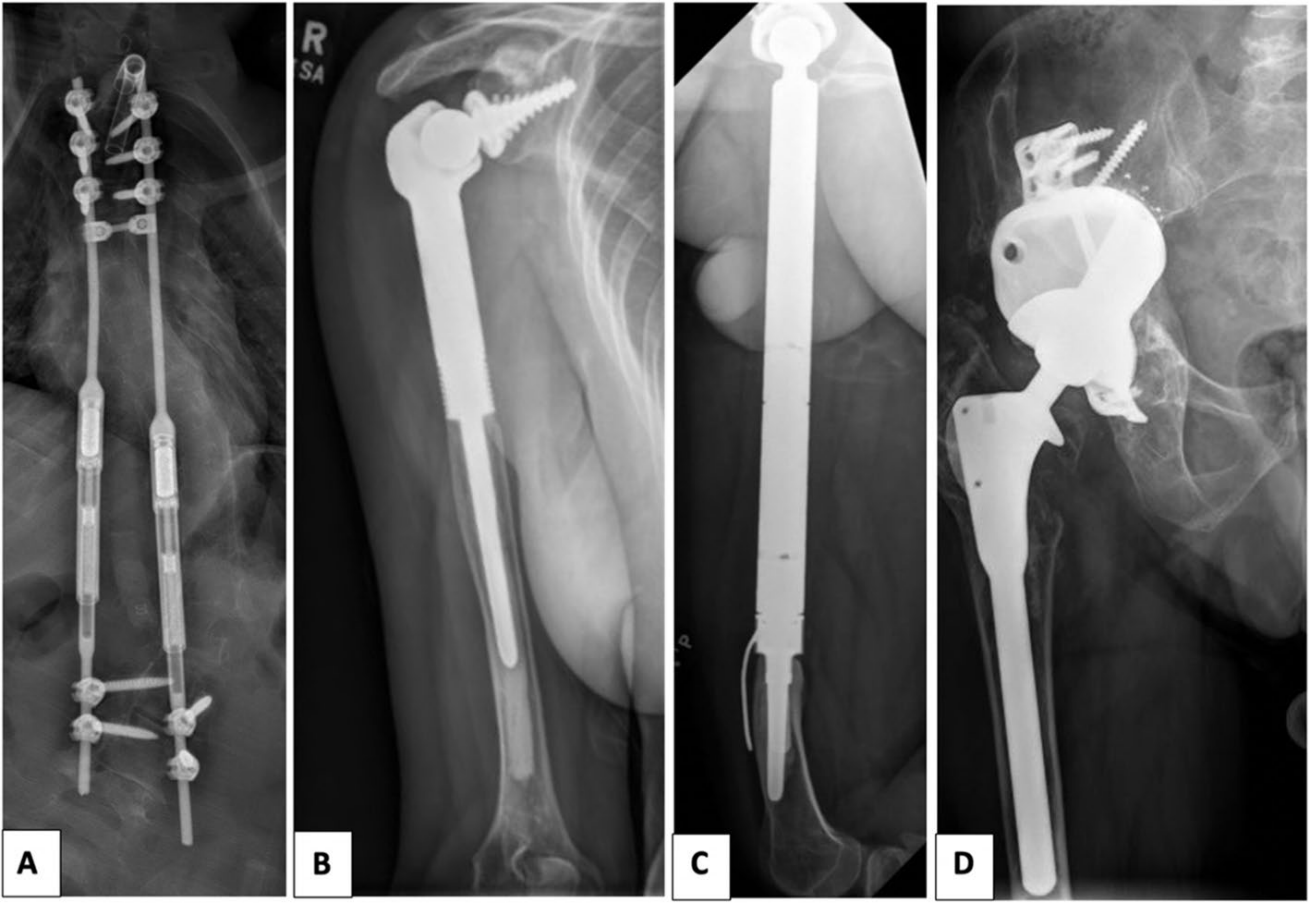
Planar frontal radiographs of the different implant types involved in this study. The radiographs were taken prior to blood samples collection. A Double spine rods construct; B Humeral tumour replacement; C Hip tumour megaprostheses; D 3D-printed customised massive acetabular defect implant
